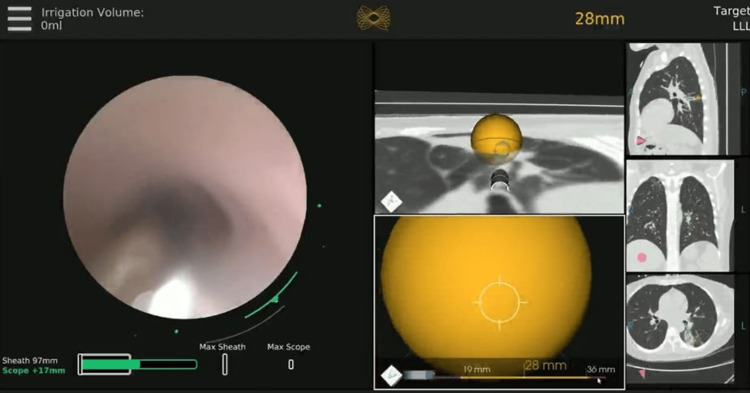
Robotic-assisted bronchoscopy for first-pass sampling of peripherally located lung nodule Image on the left side displays the real-time scope view with the ability to see our instruments in the periphery to ensure sample quality in the right upper lobe apical subsegmental bronchus.  Middle images show the generated pathway to the right upper lobe nodule and display targets straight ahead. CT images on the right side show the corresponding sagittal, coronal, and axial views of the tumor in the right upper lobe.
